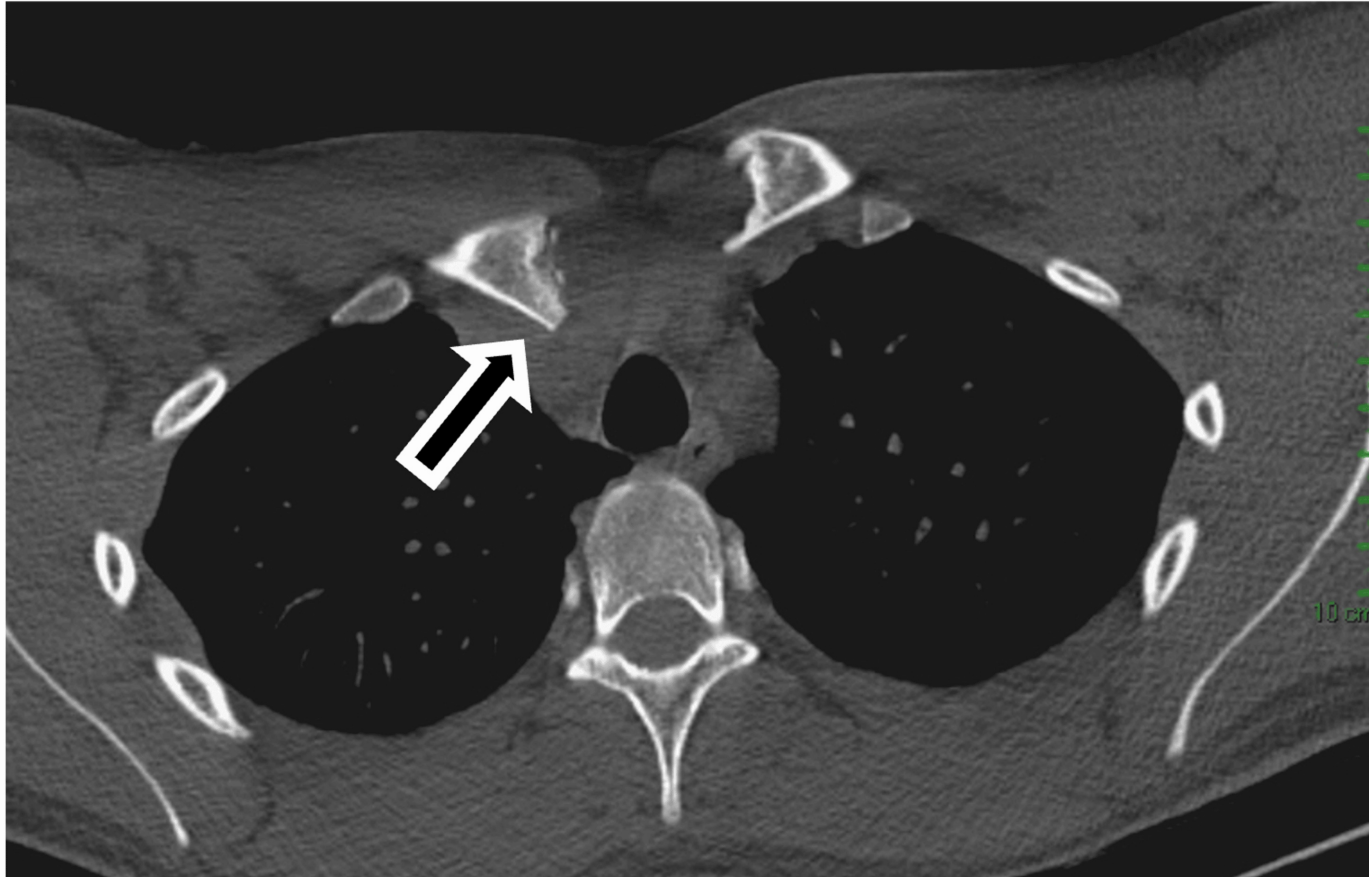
Posterior sternoclavciular joint dislocation Axial computed tomography scan of both sternoclavicular joints taken in the emergency department at initial admission. This demonstrates a posterior dislocation of the left sternoclavicular joint (black arrow).
